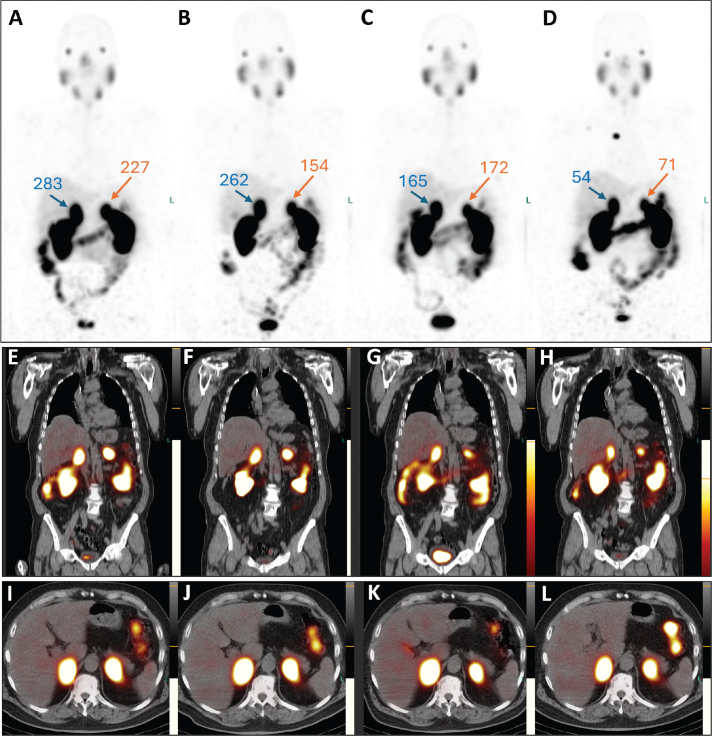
Post-therapeutic SPET/CT images. Maximum intensity projection images: A, July 2023; B, September 2023; C, October 2023; D, December 2023. Coronal: E, July 2023; F, September 2023; G, October 2023; H, December 2023. Transversal: I, July 2023; J, September 2023; K, October 2023; L, December 2023. Throughout the therapy cycles, bilateral adrenal masses show a decrease in both PSMA uptake and lesion size. The tumor-to-background ratio for each adrenal lesion, calculated based on the mean counts of radiotracer activity (as described in Figure 3), is provided.
